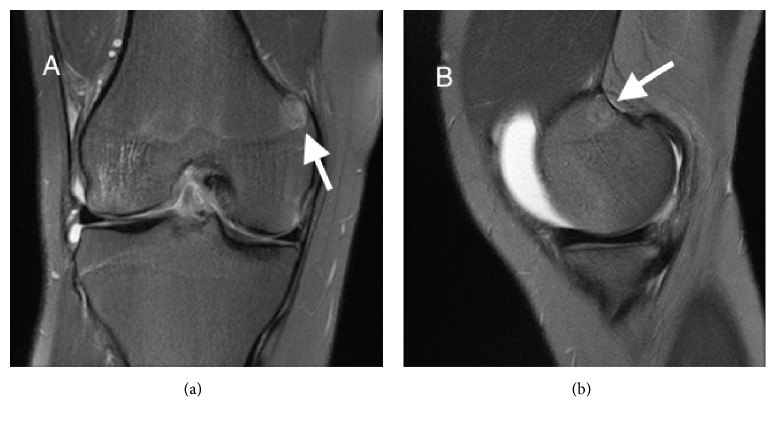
MRI coronal (a) and sagittal (b) T2 fat saturation images at initial presentation for right knee pain status after hyperextension in 2011. The arrows indicate a round T2 heterogeneous but mostly hypointense subcortical intraosseous lesion arising in the posterior aspect of the medial distal femoral metaphysis. There was also a complete ACL tear and knee joint effusion.
